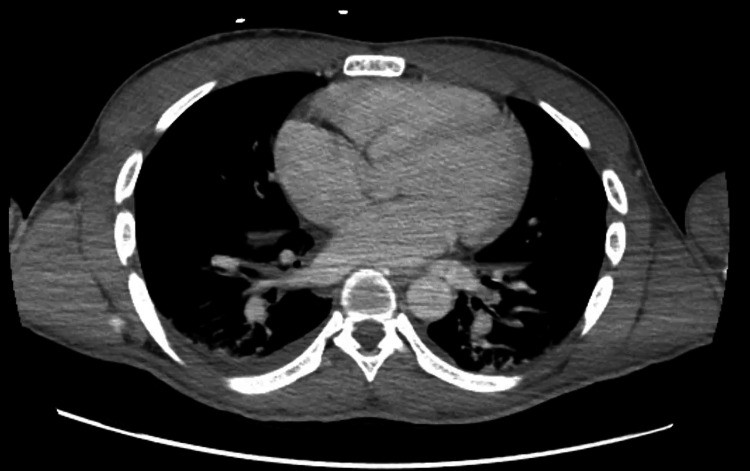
CT scan of the chest This image shows interstitial edema in both lungs and bilateral pleural effusions.
